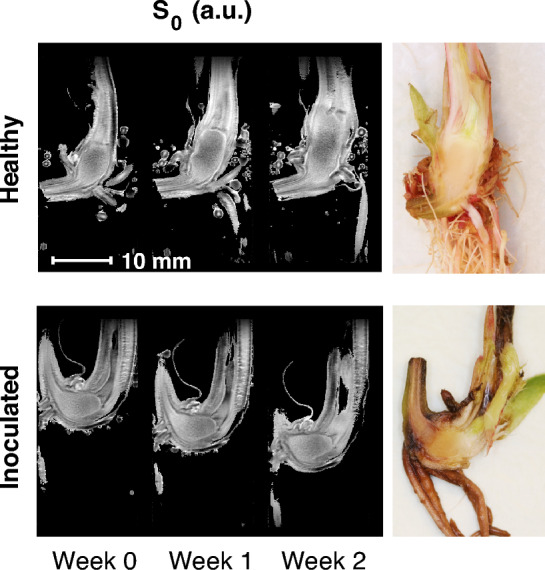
Example of proton density maps. Normalized average proton density $$S_{0}$$ maps of healthy and inoculated strawberry crowns during the two-week development period after inoculation. Average proton densities were calculated from $$S_{0}$$ maps obtained together with $$T_{1}$$ and $$T_{2}$$ relaxation time maps. Differences in the visibility of the stolon and root structures between time points are due to slightly different MR image slice positions. The representative examples here are #4 from healthy and #1 from inoculated plantlets presented in Supplementary Fig. S1 online. Values are presented in arbitrary units (a.u.) and the values from surrounding water have been omitted (black).
